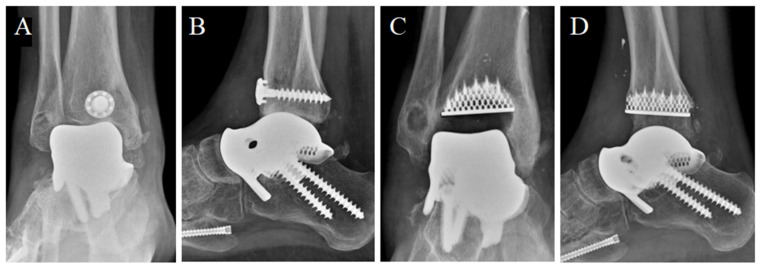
Two-year post-surgery X-ray of the implant from antero-posterior (A) and latero-lateral (B) projections. Articular space narrowing and osteophyte formation (compared with Figure 7) can be observed (A,B). The total talar replacement after revision surgery from antero-posterior (C) and latero-lateral (D) projections. *Image courtesy of Dr. Paola Verde Aerospace Medicine Department, Aerospace Test Division, Pratica di Mare, Rome, Italy.*
